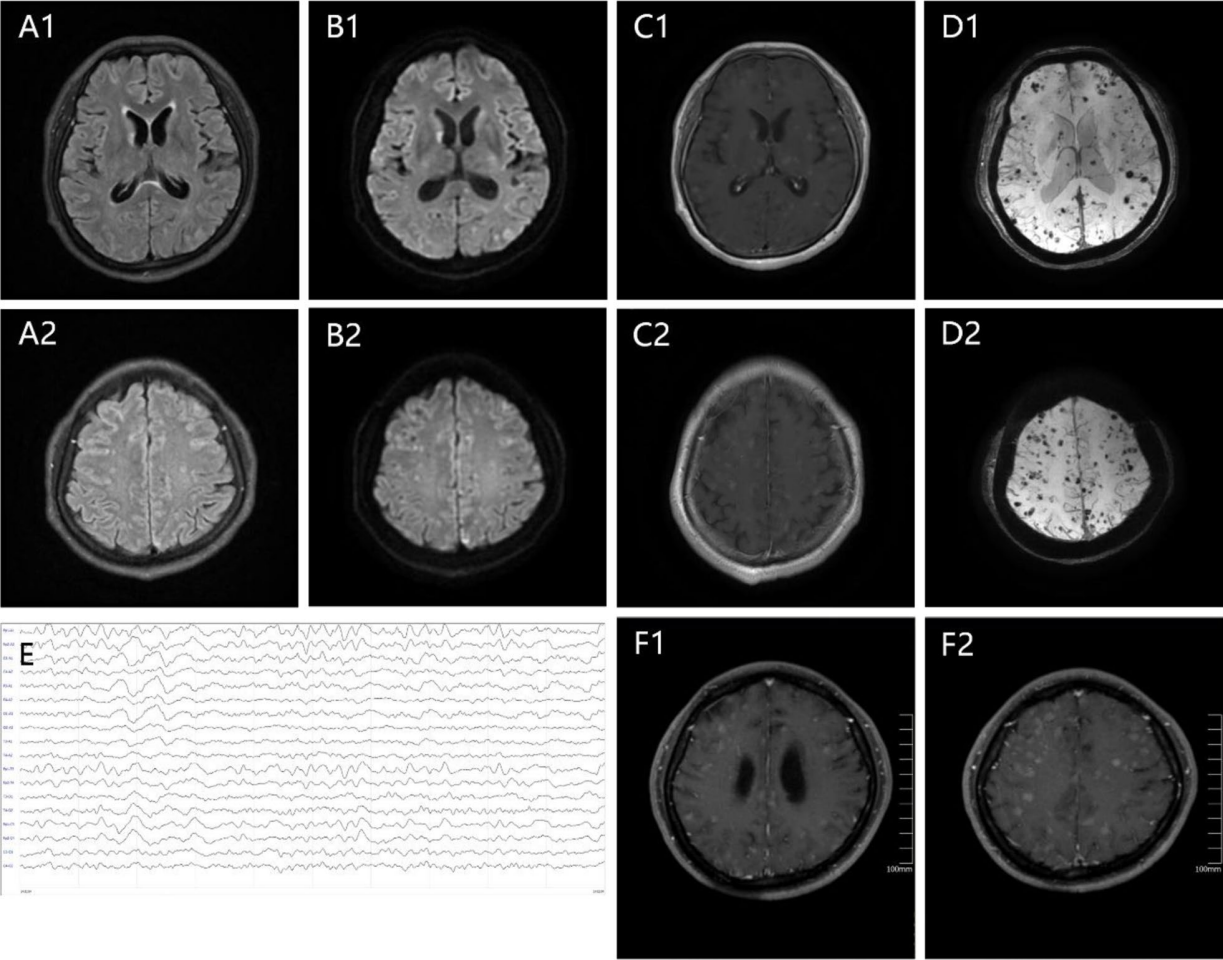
A–D multiple round abnormal signals distributed in the bilateral frontal, parietal, temporal, and occipital lobes; semi-oval center; basal ganglia region; brainstem; and cerebellum, with diameters of approximately 3–5 mm. A1–A2: T2 flair showing longer T2 signals; B1–B2: Diffusion-weighted imaging (DWI) showing some lesions with restricted diffusion; C1–C2: lesions showing nodular or ring-like enhancement; D1–D2: Susceptibility-weighted imaging (SWI) showing multiple cerebral microbleeds; E: 24-hour video electroencephalogram showing widespread slow waves; F: T1 enhances showing lesion progression compared with the previous images
